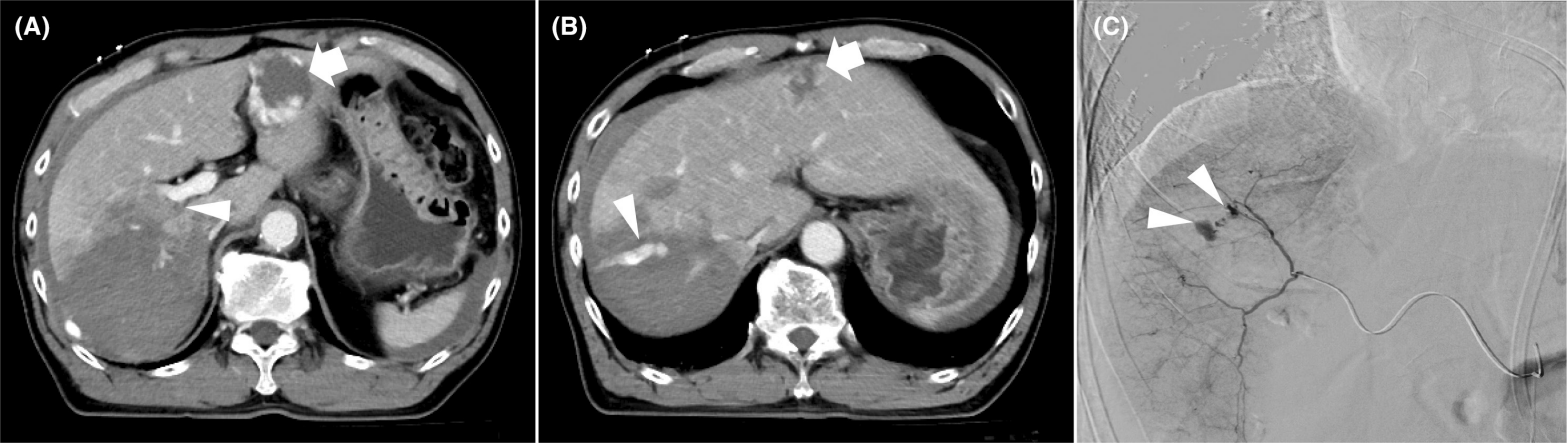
Imaging on the day of admission. (A) Contrast‐enhanced CT scan showing loss of contrast effect in the posterior section of the liver and occlusion of the posterior portal venous trunk (arrowhead). (B) Extravasation from the segment VII artery can be seen. Arrows indicate hemangiomas in segment IV, as noted initially (A, B). (C) A hepatic angiogram confirmed active segment VII artery bleeding (arrowhead). The bleeding artery was embolized.
